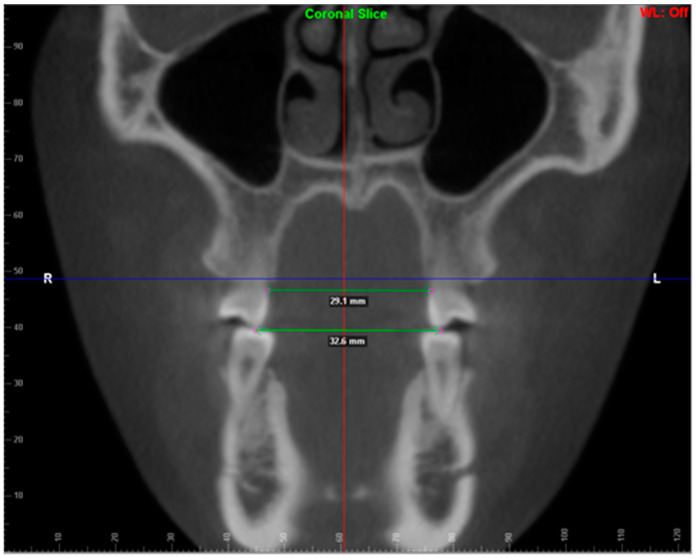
CBCT transverse sectional image shows the transverse measurements at the maxillary first molar level; from the lingual cemento-enamel junction (gingival margin) and between the tip of the lingual cusp.
